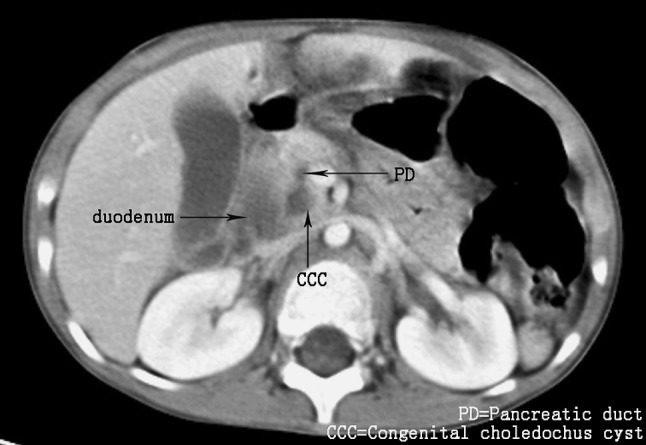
Abdominal enhanced computed tomography, showing convergence of the dilatation of the common bile duct and pancreatic duct
